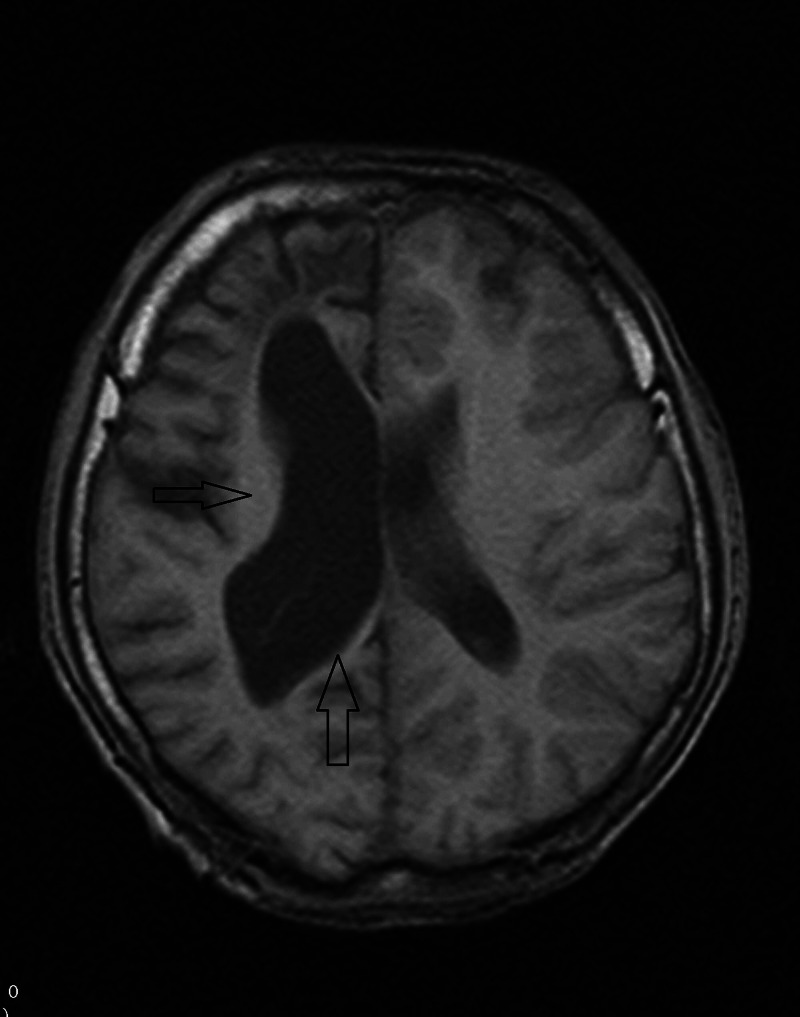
MRI (T1W) showing atrophic right cerebral hemisphere with dilatation of the right lateral ventricle and extra-axial cerebrospinal fluid (CSF) spaces (black arrows)
